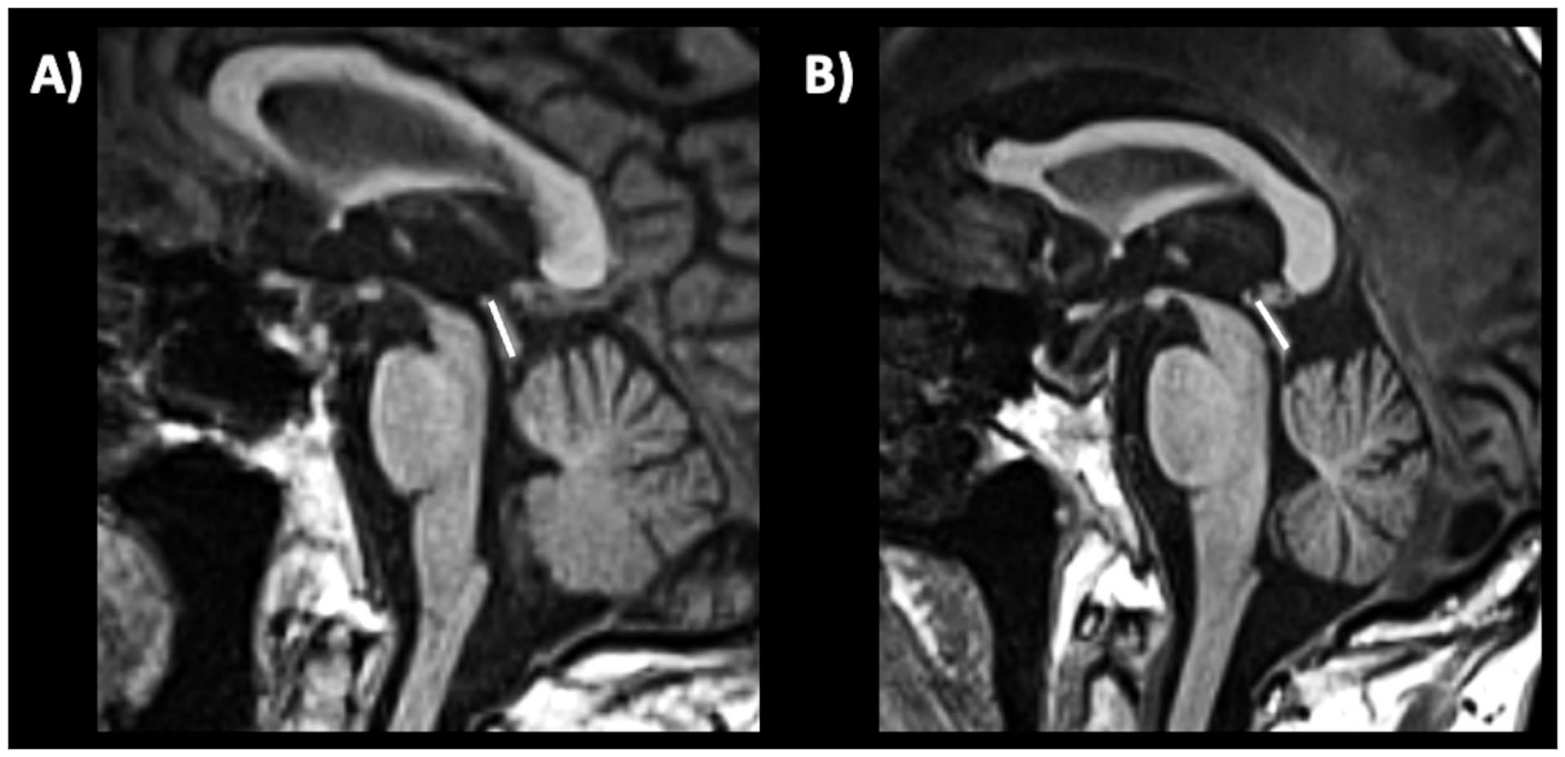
A 3T brain T1-weighted midsagittal MR image showing how to measure the length of the tectal plate, in a patient with progressive supranuclear palsy (A) and in a patient with Parkinson’s disease (B).
